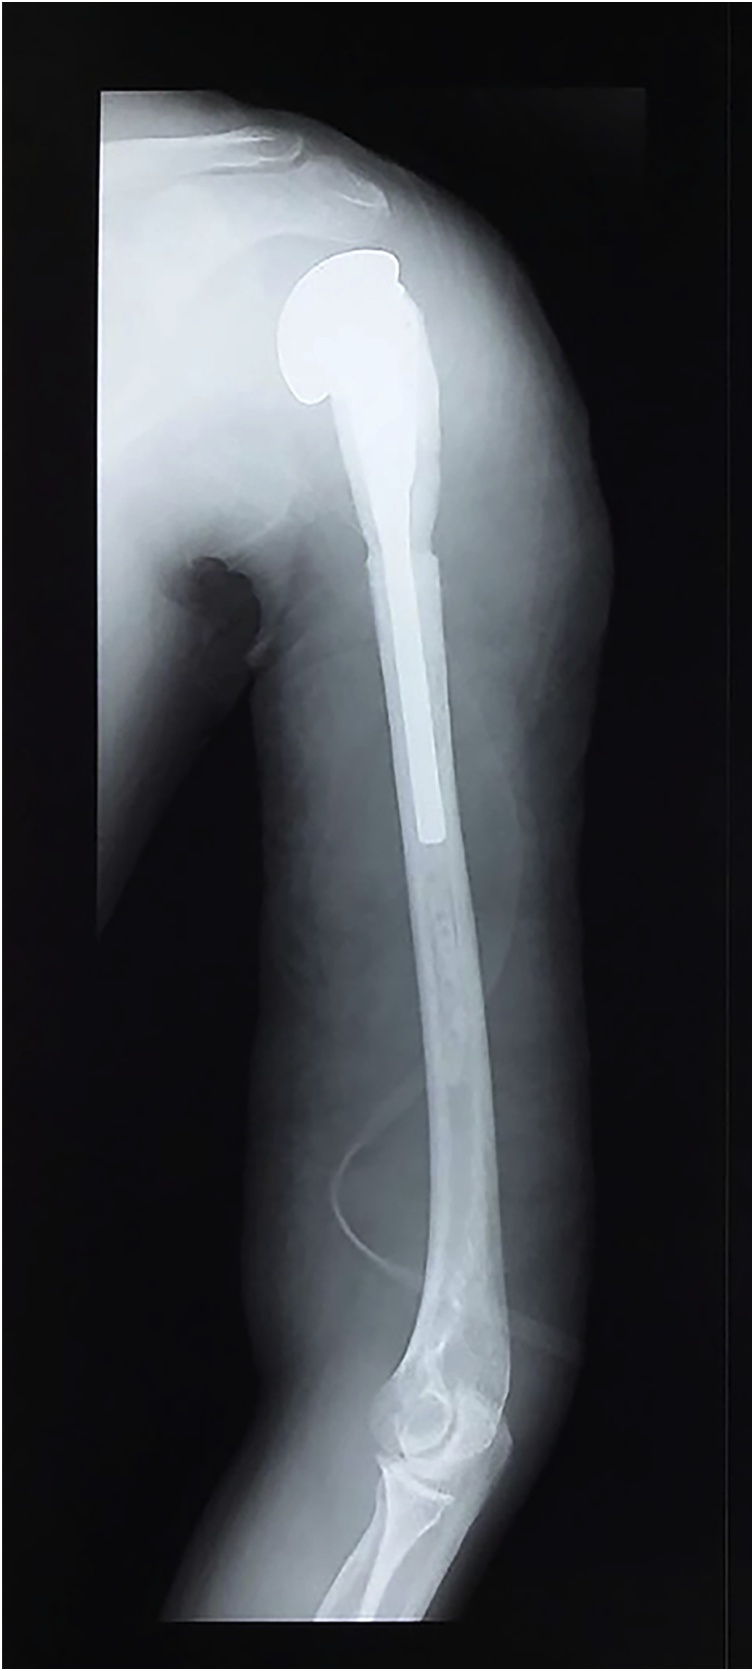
Post-operative radiograph of the left shoulder.
